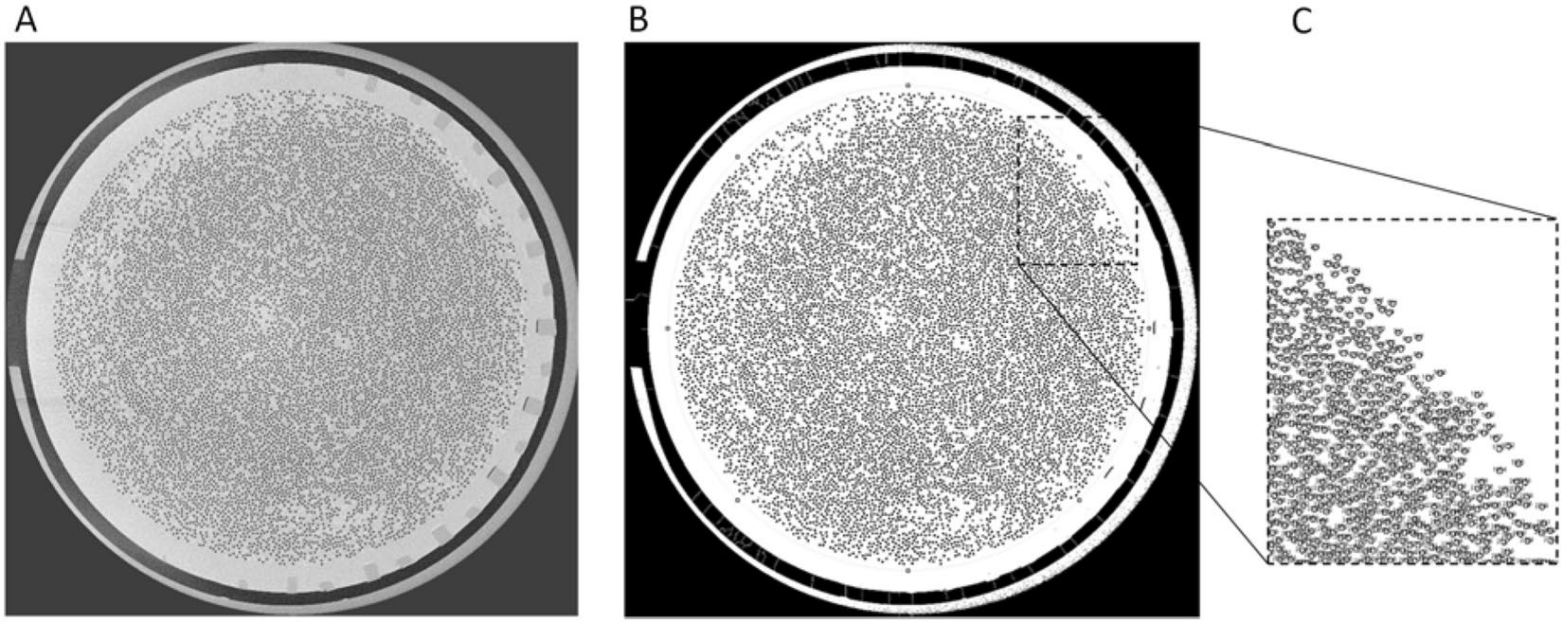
CT image of cross-section (panel A). Optimization of image brightness and selection of the region of interest (ROI) bordering the entire fiber bundle (panel B). Zoomed inset of counted fibers (panel C).
